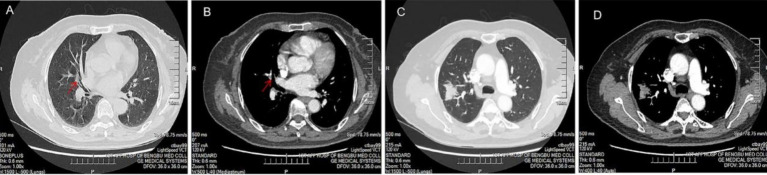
Coexisting pulmonary sclerosing pneumocytoma (PSP) and lung adenocarcinoma on CT. (A-D) One patient with a 7.6 mm × 6.1 mm nodule in the right middle lobe showing a marginal vessel sign; a separate upper-lobe mass was confirmed as lung adenocarcinoma.
